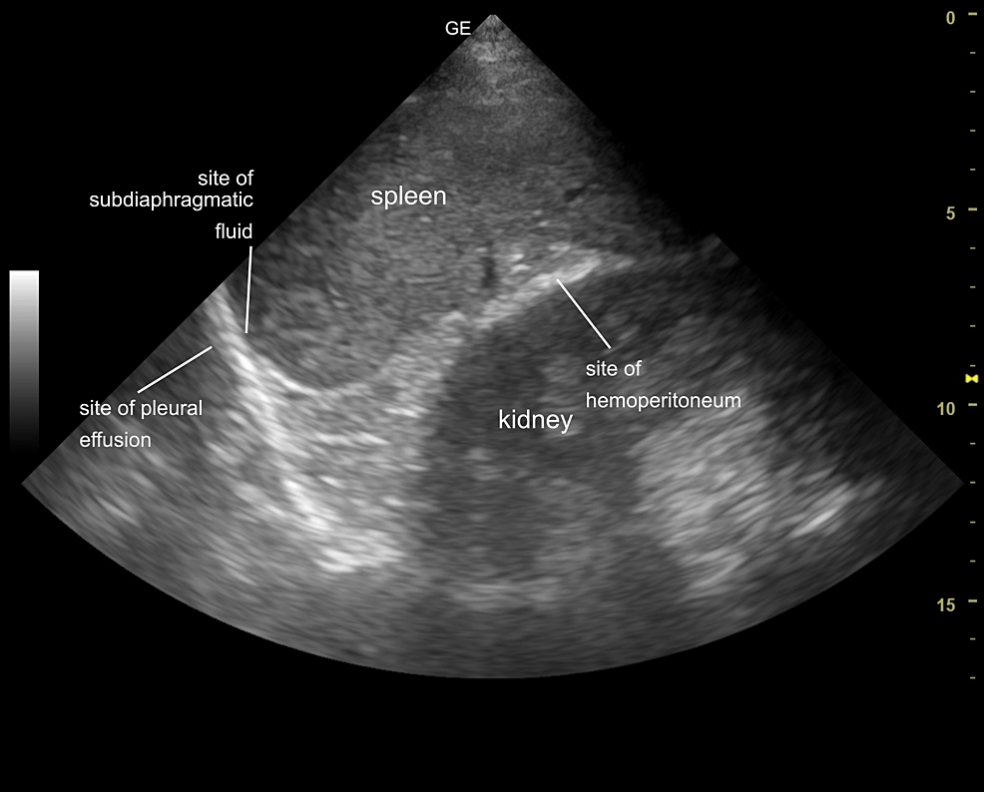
Left upper quadrant ultrasound image The gross view was correlated with the ultrasound image and the sites of potential fluid accumulation in the pleural, subdiaphragmatic, and peritoneal spaces were identified.
